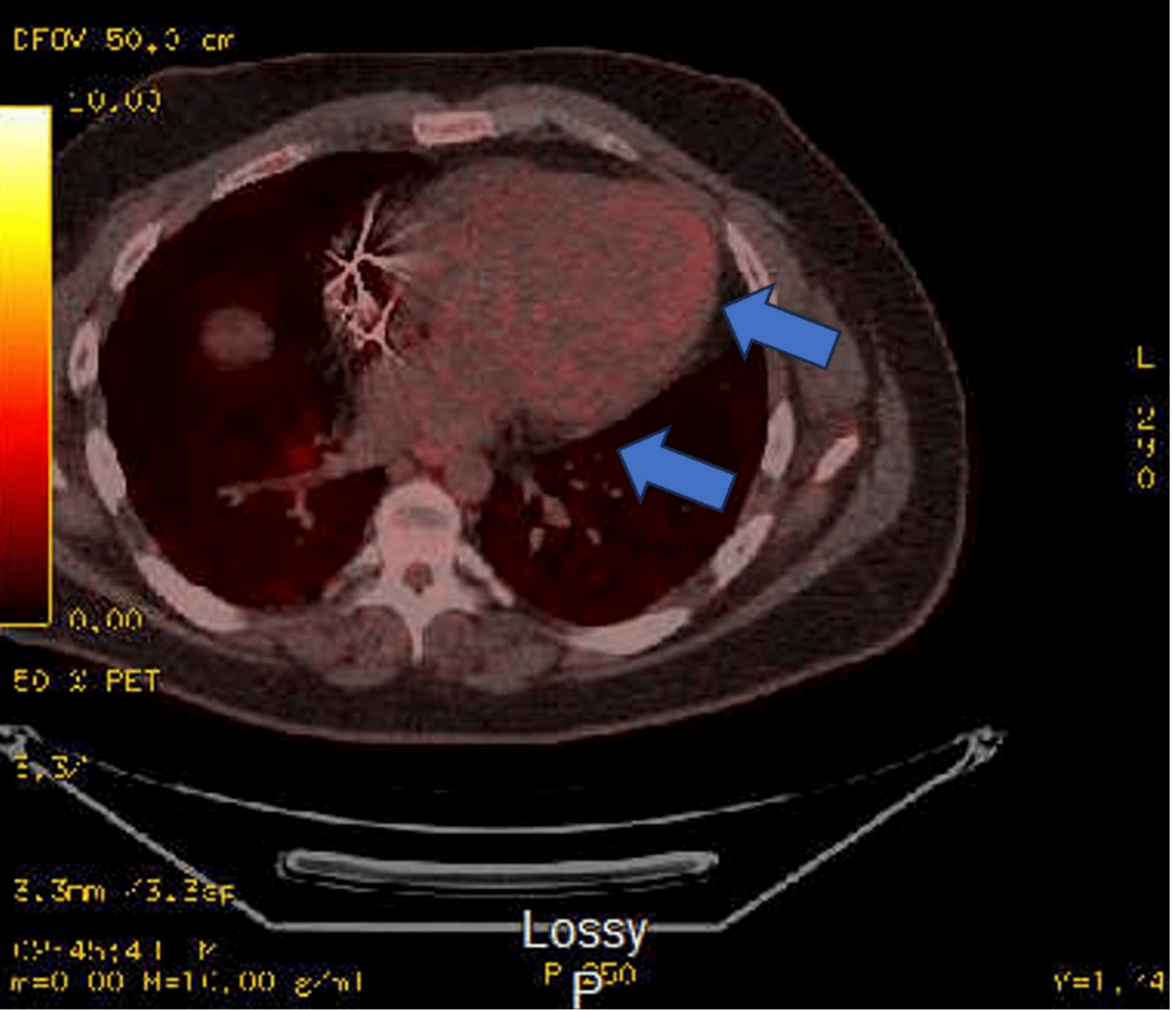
The FDG-PET myocardial metabolic imaging demonstrates focal areas of FDG uptake indicative of metabolic activity at the level of the cardiac apex, apical anterior, apical lateral, and anterolateral walls (blue arrow). A smaller region of FDG uptake is also observed in the inferolateral wall. FGD-PET: fluorodeoxyglucose positron emission tomography
